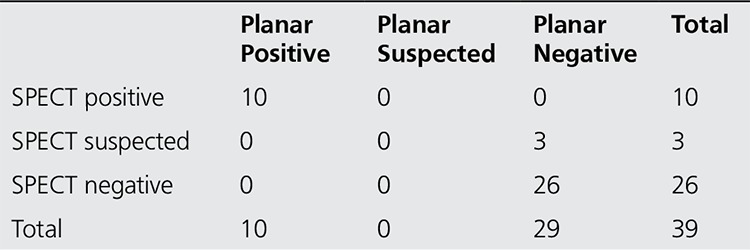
Comparison of planar with SPECT images
